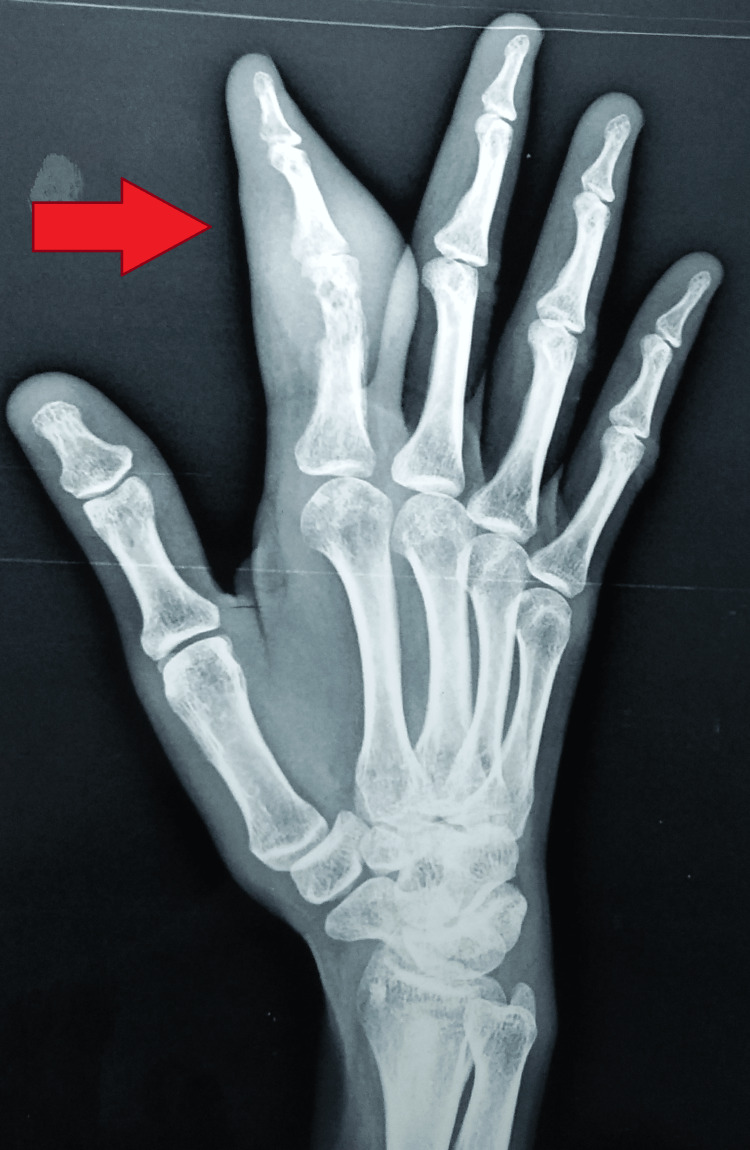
Radiograph of right hand oblique view showing cortical erosion in the distal part of proximal phalanx, and minimal periosteal reaction
